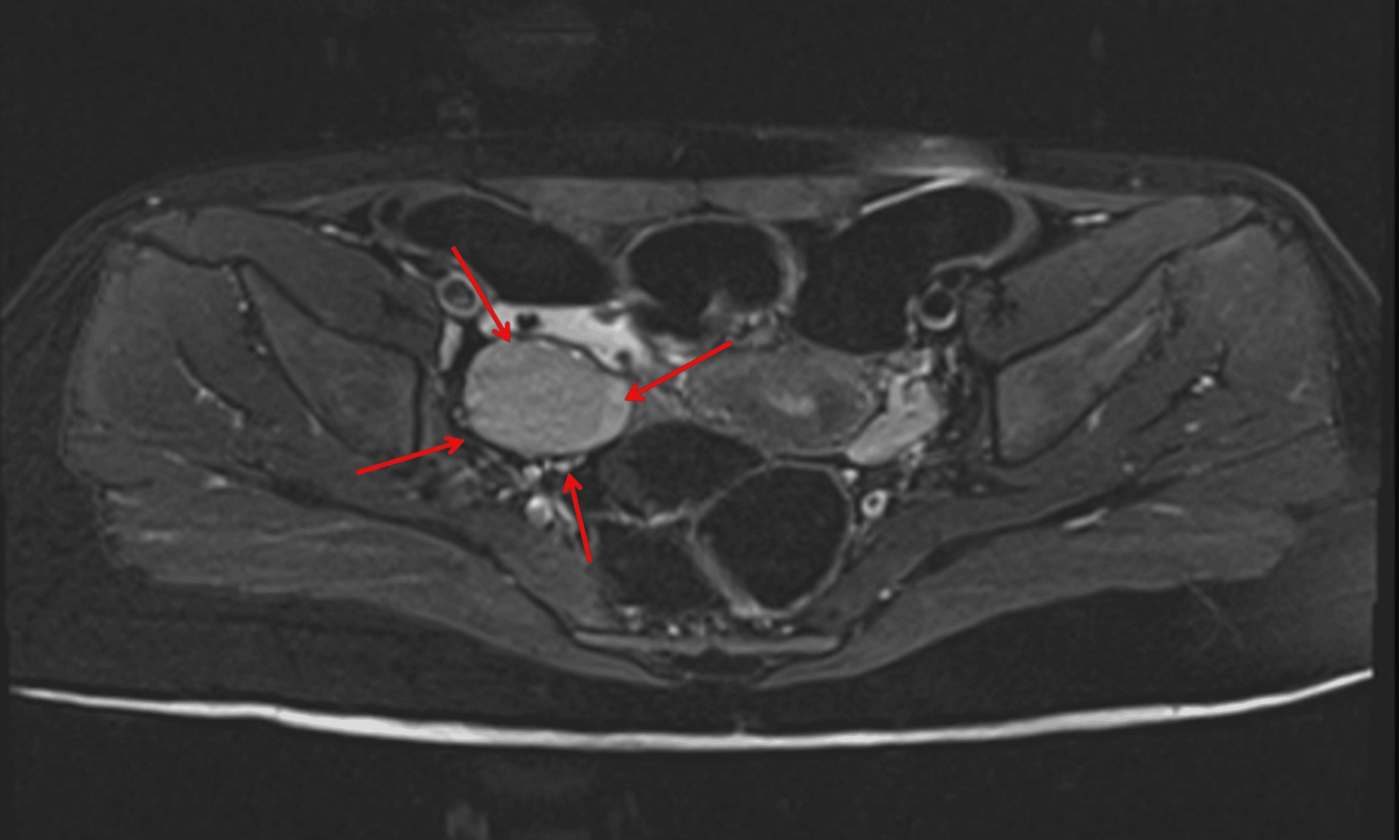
Right ovarian tumor MRI transverse image (Case 1) Transverse T2-weighted MRI showing a well-defined, approximately 3-cm right ovarian cystic lesion. Both fluid and soft-tissue components are visible, consistent with a mature cystic teratoma (dermoid cyst). The lesion is situated lateral to the uterus, reinforcing its ovarian origin.
